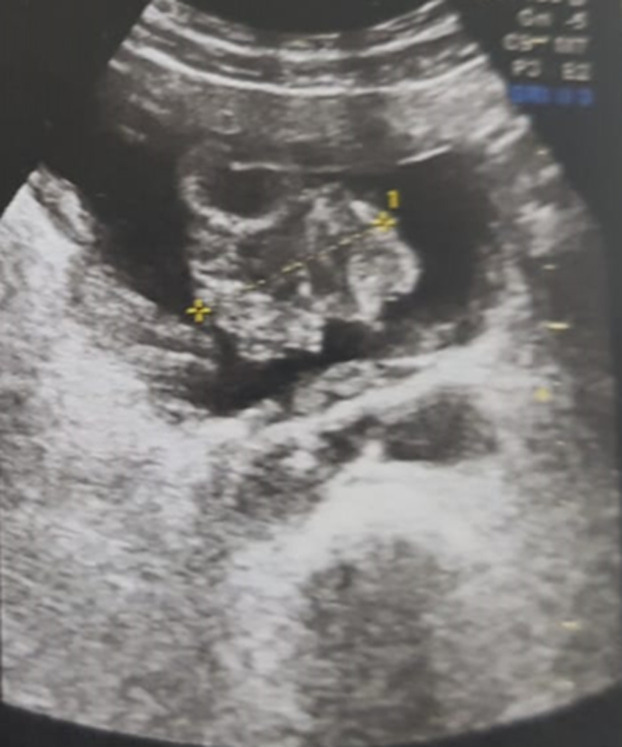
ultrasound showing a parietal mass depending on the anterior surface of the bladder
